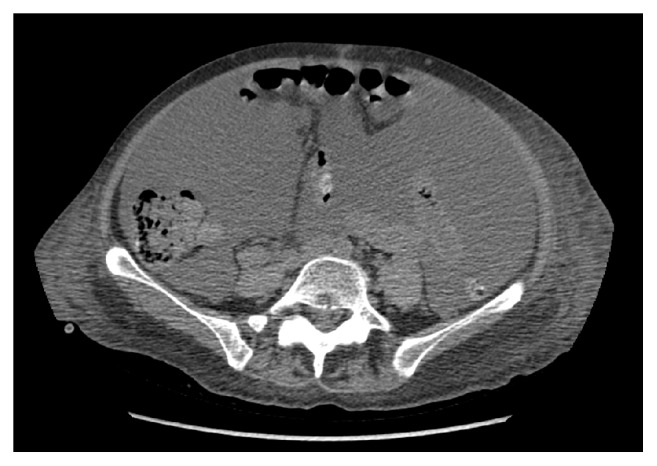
Liver metastasis (CT abdomen and pelvis, noncontrast transverse).
